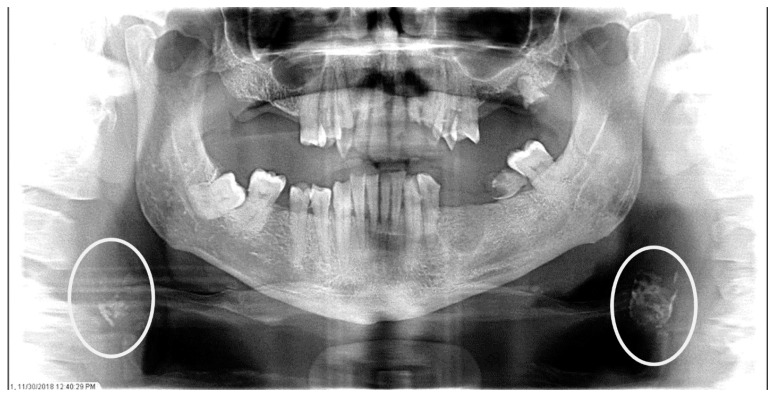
The panoramic radiograph of a 53-year-old male patient with a medical history of hypertension and hyperlipidemia showing bilateral carotid artery calcifications (CACs) during a comprehensive dental examination. The CACs are encircled by a white line.
